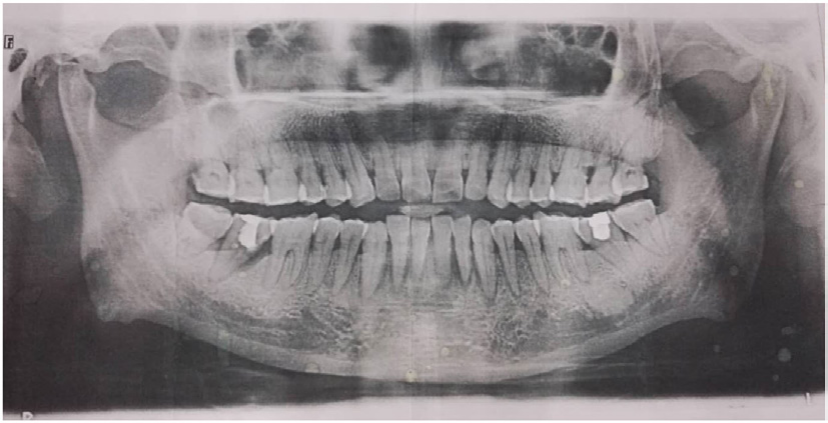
The panoramic radiography of the jaw was normal.
